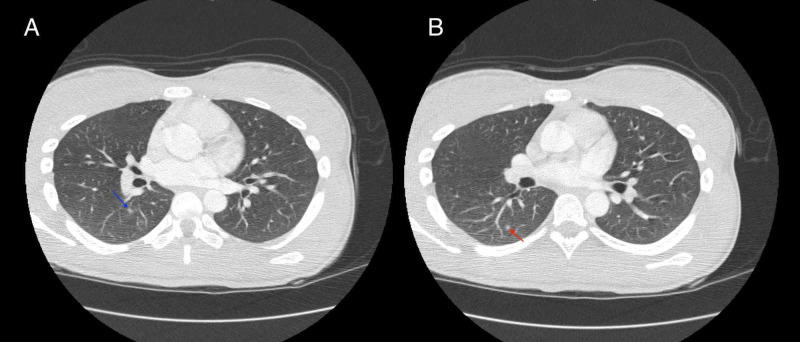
Axial CT images of the thorax. Axial CT images of the thorax demonstrate two focal areas of ground glass opacities within the superior portion of the right lower lobe. (A) The larger ground glass nodule is located posterior to the right perihilum, and measures 4.1 mm in greatest dimension (blue arrow). (B) The smaller ground glass nodule is located slightly more posterosuperiorly, and measures 3.2 mm in greatest dimension (red arrow).
